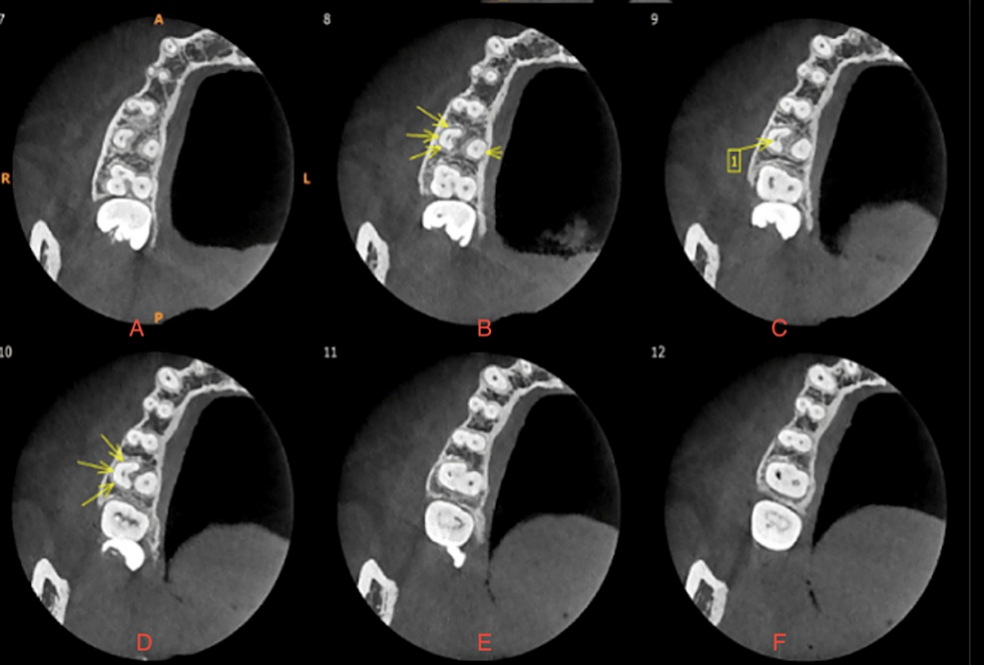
CBCT of the transverse section of teeth: (A-F) CBCT images at different levels coronally; 1: C-shaped root canal after fusion of the mesiobuccal and distobuccal roots. CBCT, cone-beam computed tomography
